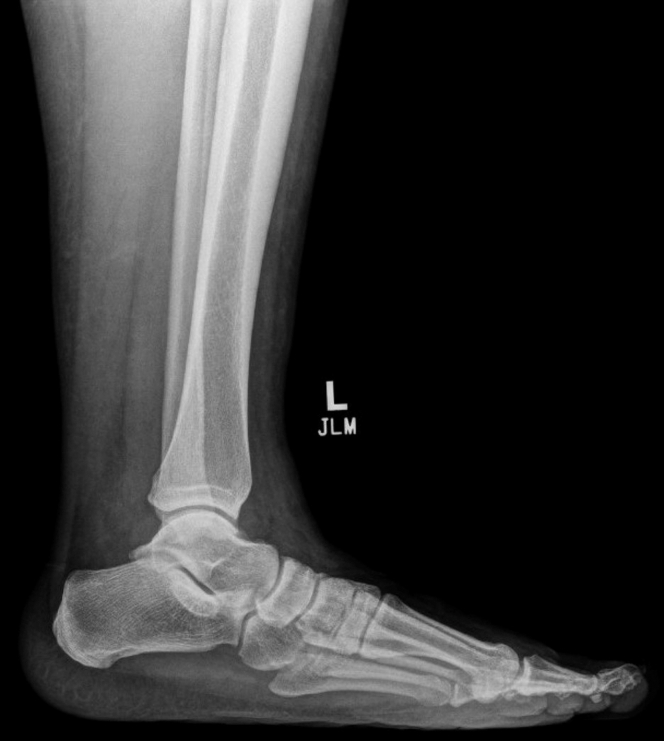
Lateral radiograph at 10-day follow-up.
